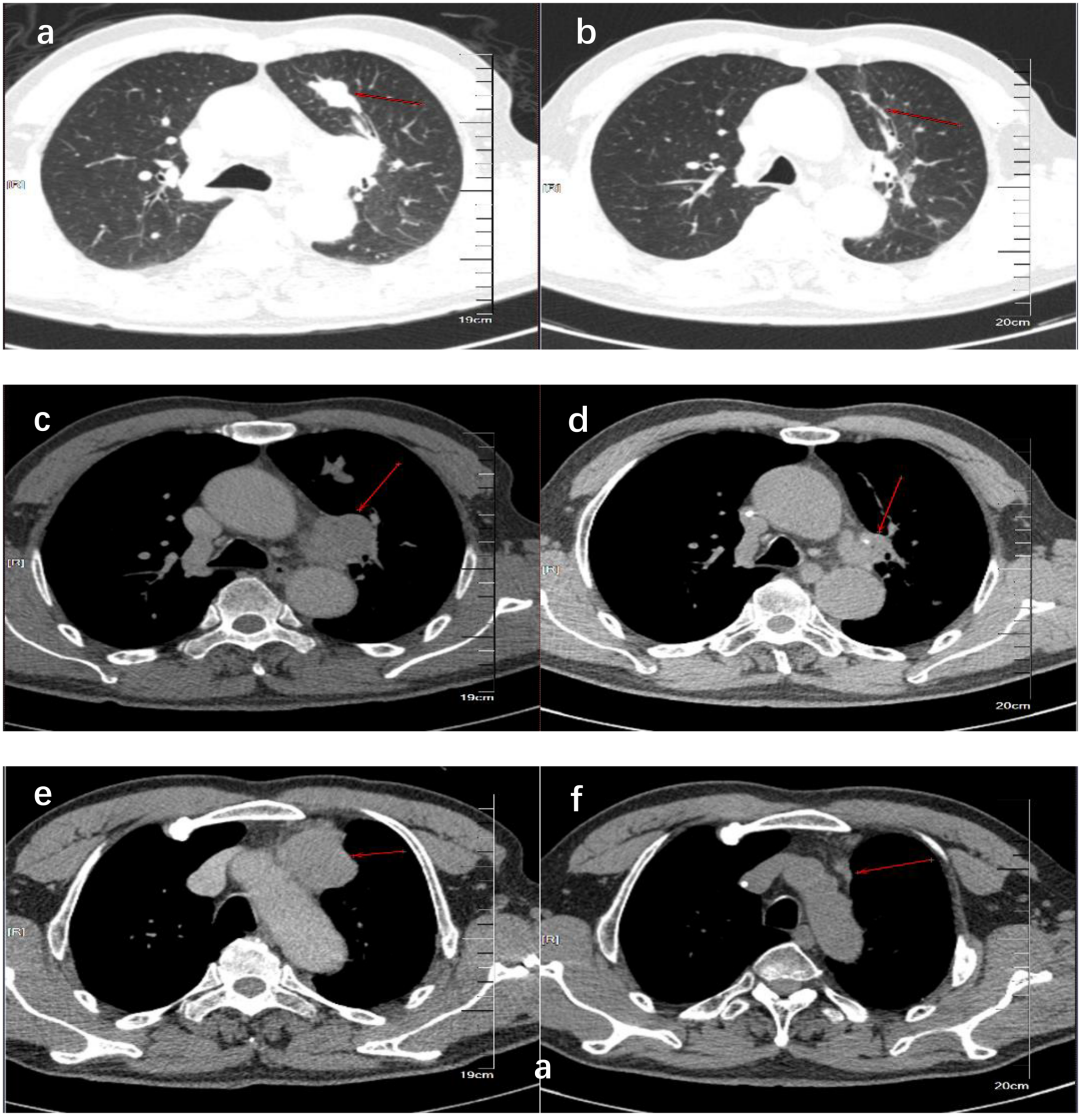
CT showed the comparison of lung lesions before (A, C, E) and after (B, D, F) the treatment of concurrent chemoradiotherapy. (A, B) show the tumor location of the primary lesion in the left upper lobe before and after treatment. (C, D) show the location of the tumor before and after treatment of the left hilar lymph node metastases. (E, F) show the tumor location before and after treatment of anterior mediastinal lymph node metastases.
